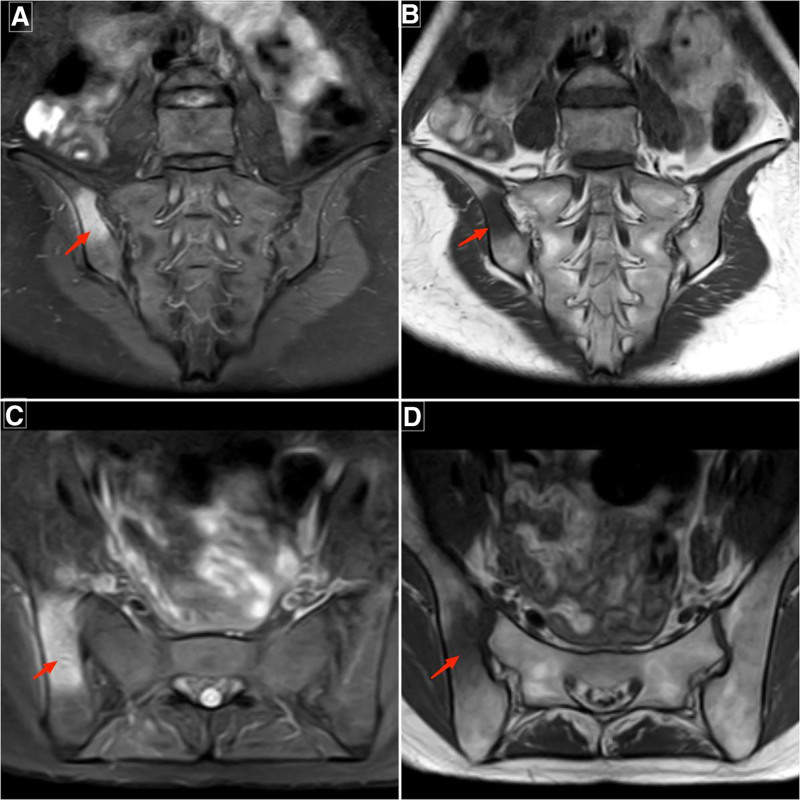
MRI of the sacroiliac joints on admission. (A and B) T2-weighted images with fat suppression show bone marrow edema, seen as patchy high signal intensity (arrows), beneath the articular surfaces of the right ilium and sacrum. Note the associated swelling of the adjacent gluteal and soft tissues, most prominent on the right.(C and D) Corresponding T1-weighted images demonstrate low signal intensity (arrows) in the same regions. The right sacroiliac joint space is narrowed. In contrast, the left sacroiliac joint appears normal in alignment, joint space width, and signal characteristics. MRI = magnetic resonance imaging.
